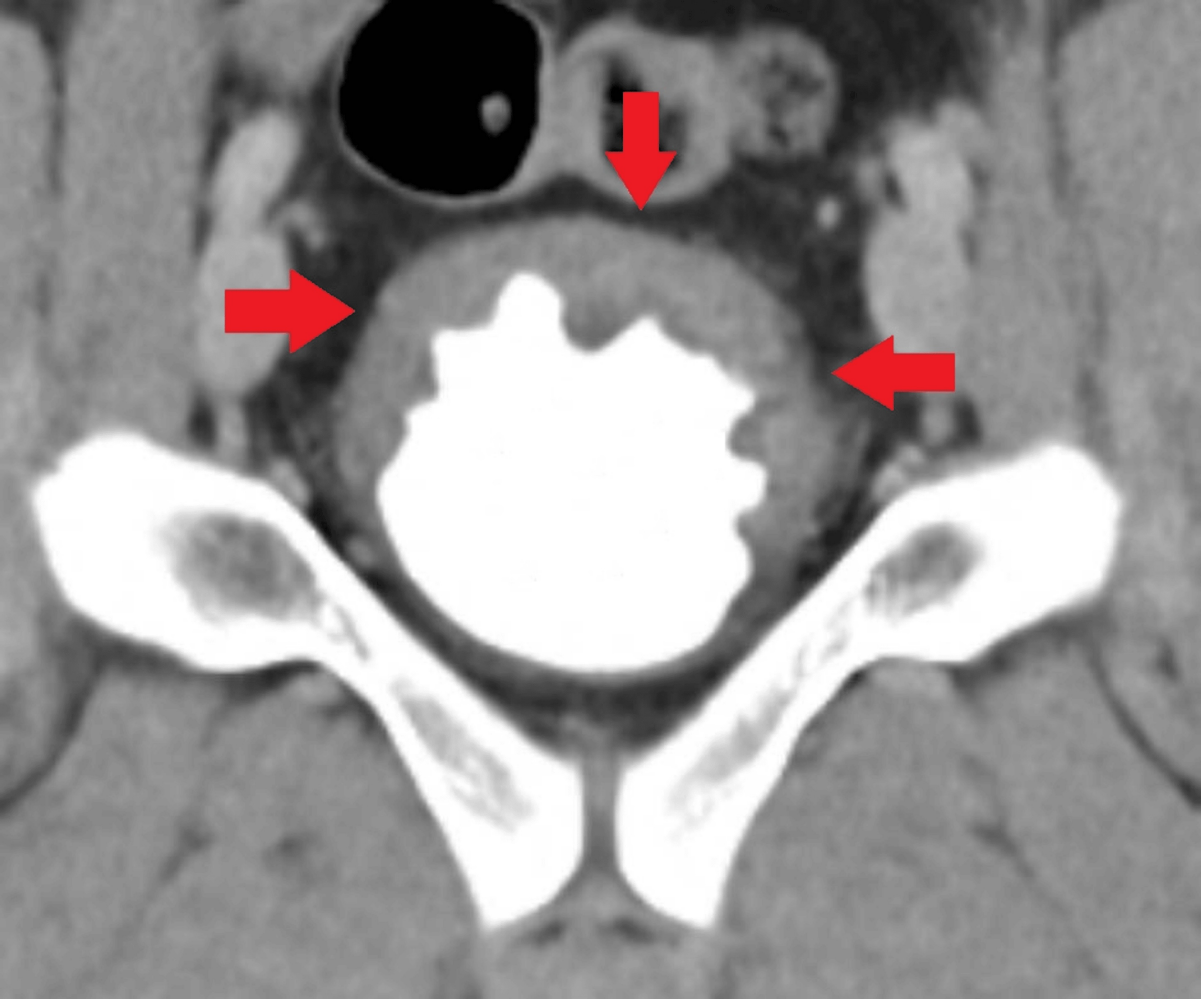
View of the partial bladder wall thickening in the coronal plane of a computed tomography scan, including the urographic delayed phase. Red arrows: affected area
